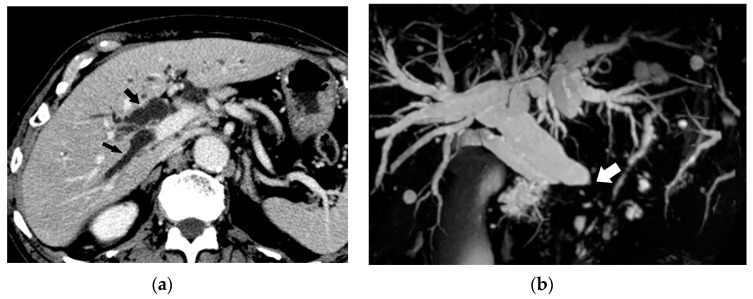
Obstructive jaundice (intra- and extra-hepatic bile duct dilatation) observed in a 77-year-old man. (a) In the portal venous phase on contrast-enhanced CT, a dilated linear hypodense structure (arrows) is observed along the portal vein in the hepatic hilar area. (b) On MRCP, common bile duct obstruction caused by common bile duct carcinoma is observed (arrow). The upstream dilated bile ducts gradually taper as they move toward the peripheral part of the liver.
